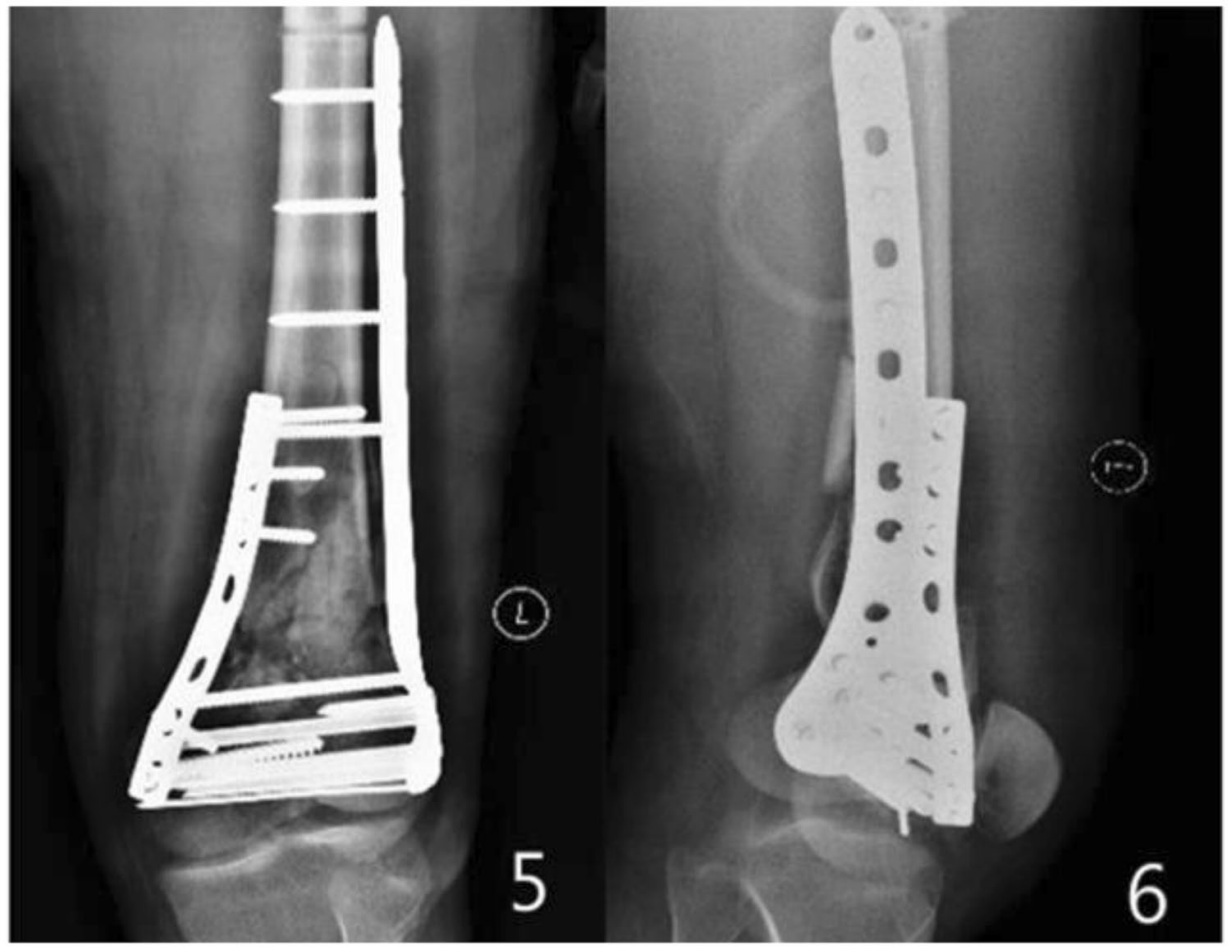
5, 6 the X-ray of the femur, taken two days after operation.
